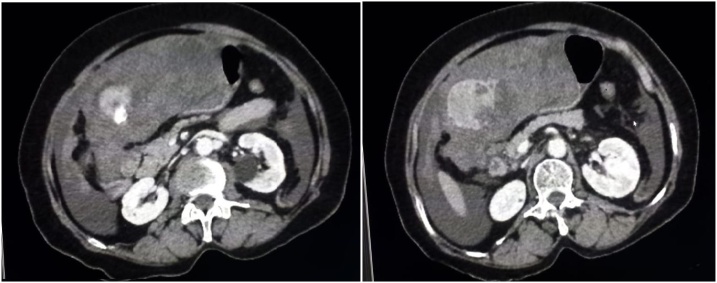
CT scan sections showing a rounded 37.44 mm segment III formation with an heterogeneous enhancement and individualization of an arterial blush with left portal time and hemoperitoneum of high abundance.
